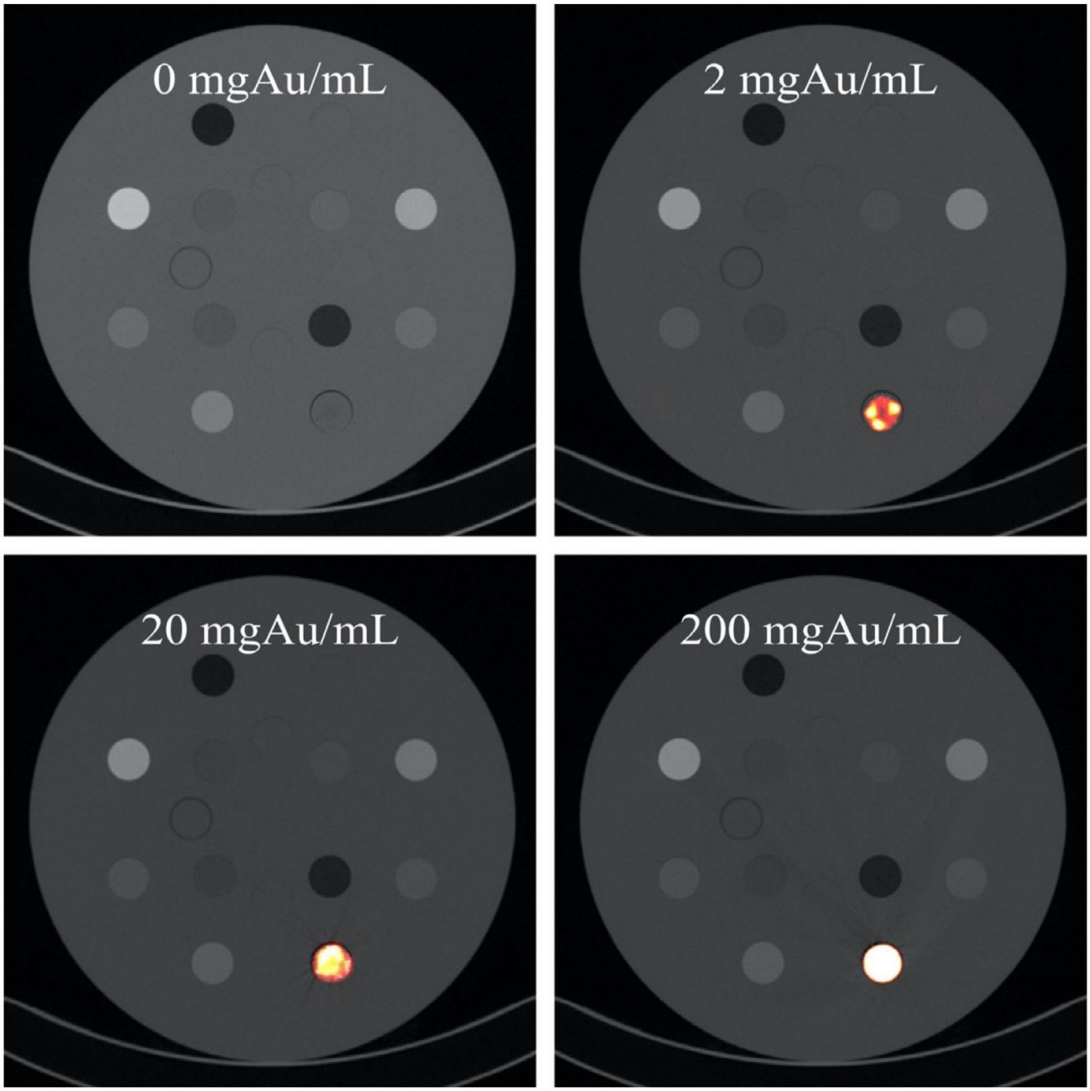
Computed tomography images of AuNPs at 0, 2, 20, and 200 mgAu/mL.
